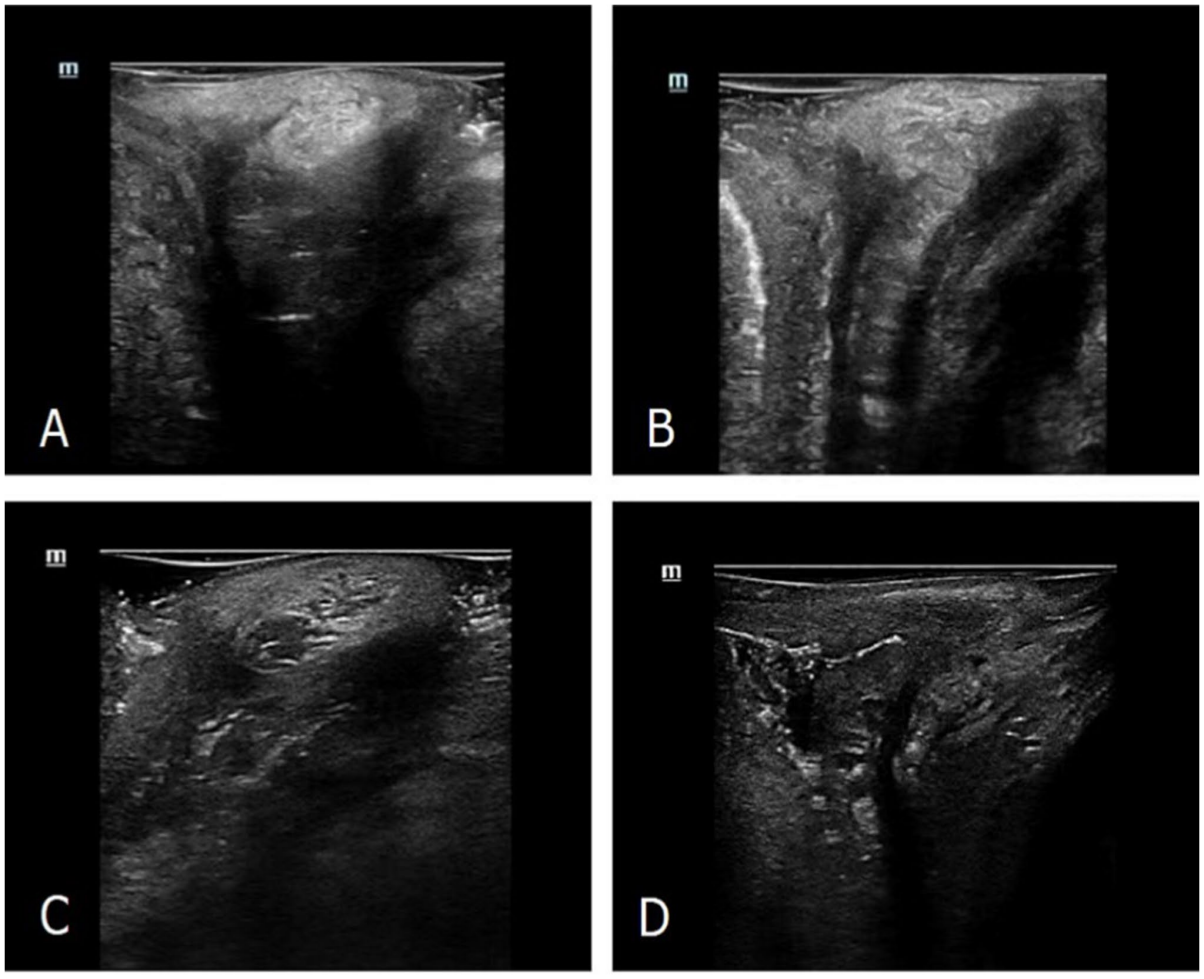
Ultrasonographic manifestations of the PB on high-frequency ultrasound. (A) The PB morphology of representative nulliparous women detected by ultrasound. (B) The PB morphology of representative parous and POP women detected by ultrasound. (C) The PB morphology of representative postpartum women detected by ultrasound. (D) The ultrasonic image of very few postpartum women with severe perineal laceration.
